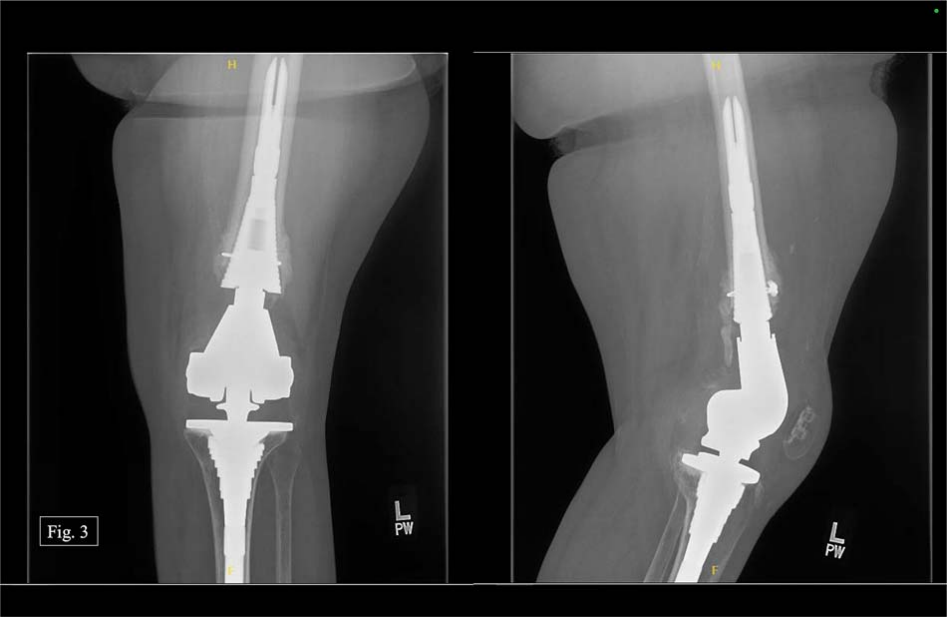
Left, 5 month postoperative AP and lateral X-rays.
